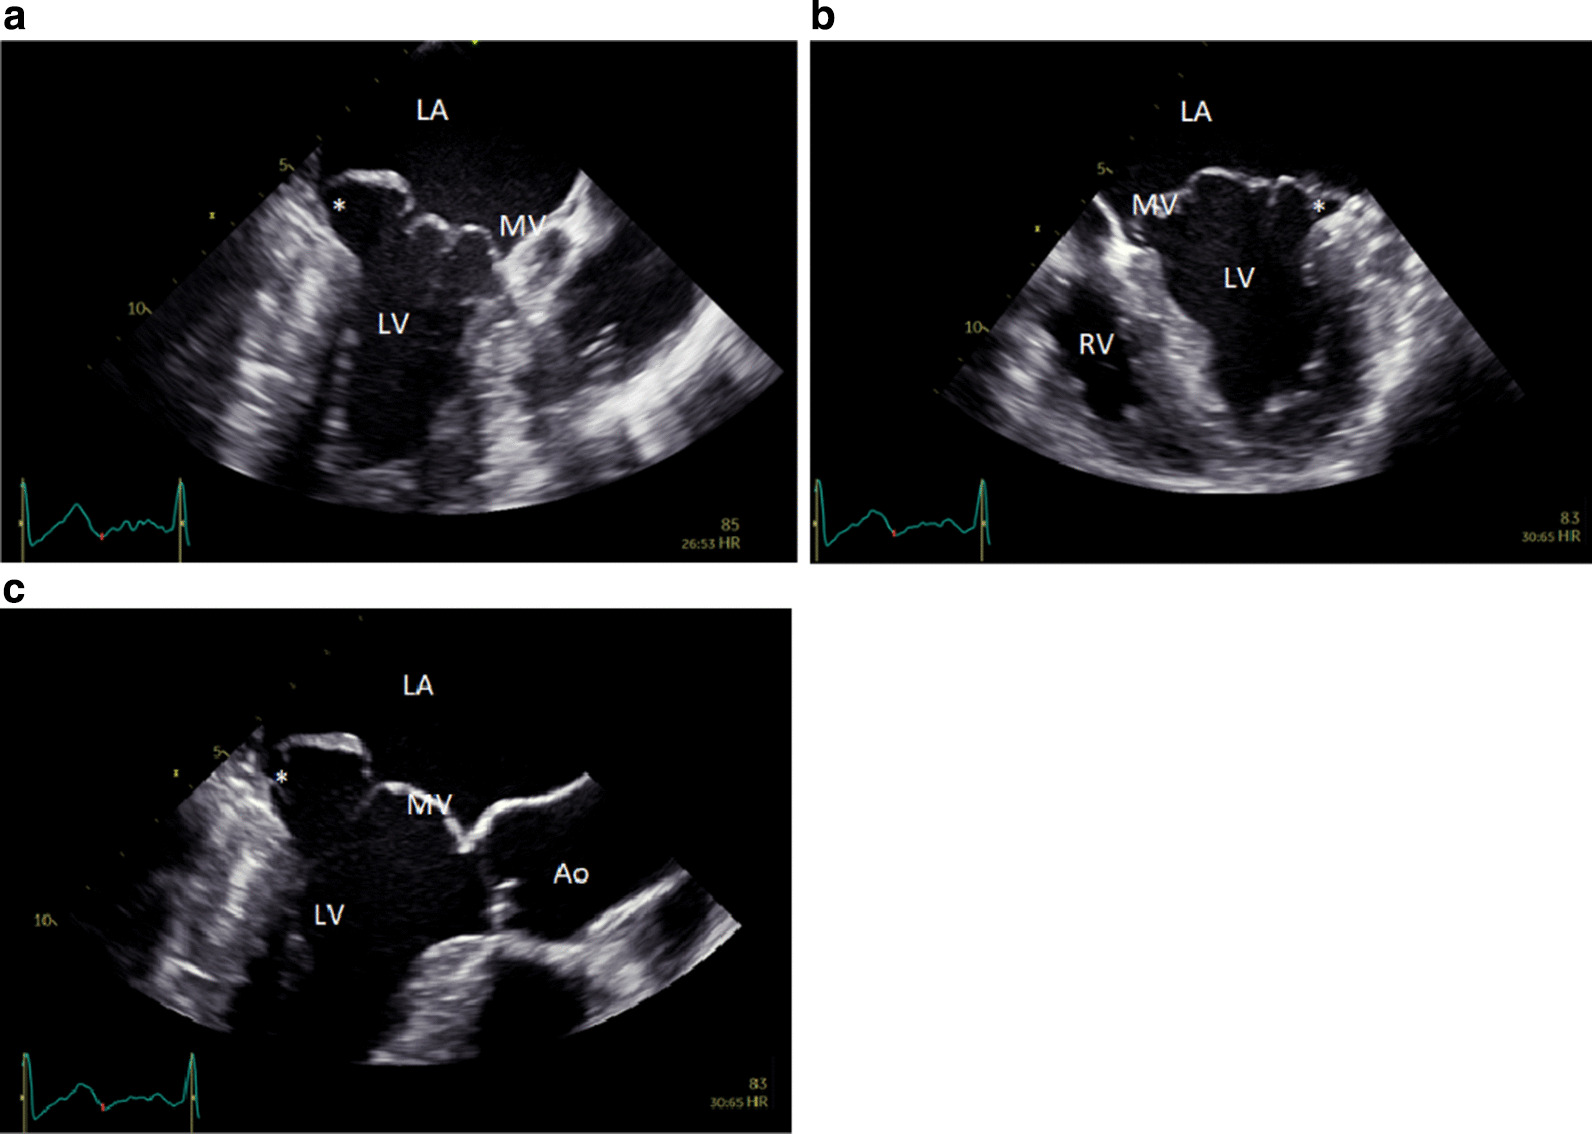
Transoesophageal echocardiography imaging of mitral annular disjunction. Mitral annular disjunction (*) as seen during ventricular on transoesophegeal echocardiography in the apical two chamber view (a), apical four chamber view (b) and apical three chamber view (c). *Ao* aorta, *LA* left atrium, *LV* left ventricle, *MV* mitral valve, *RV* right ventricle
